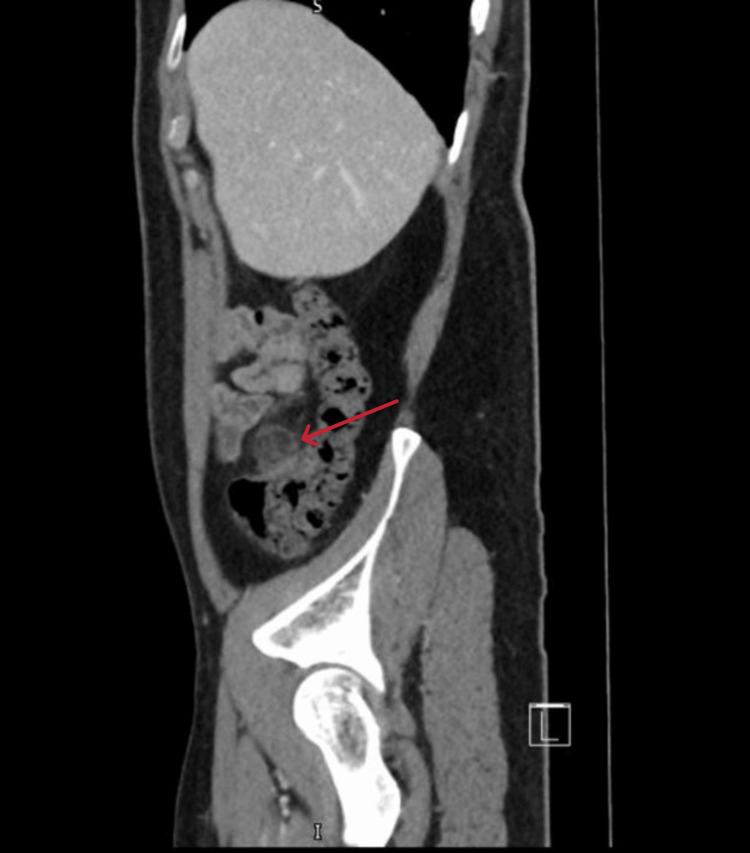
Coronal computed tomography image of epiploic appendagitis The image shows an ovoid fat-density lesion adjacent to the junction of the descending and sigmoid colon (red arrow), with surrounding inflammatory fat stranding and thickened peritoneum, consistent with epiploic appendagitis.
